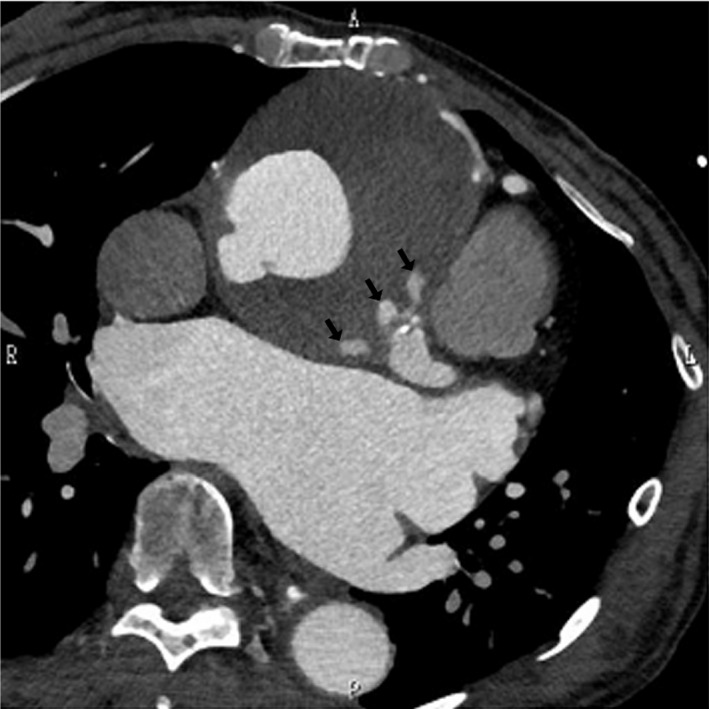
Chest CT before the fourth operation. It showed a pseudoaneurysm with leakage (black arrows) from the anastomotic site of the left coronary artery.
